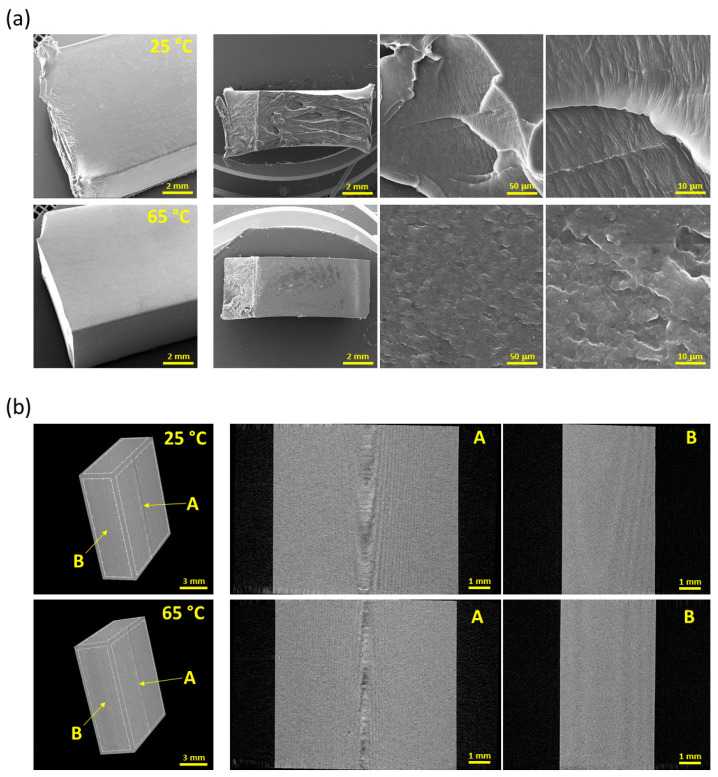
Morphology characterization imaging: SEM images showing the surface morphology of the samples on the left and the fracture morphology following the Charpy impact test at various magnifications on the right−(a) and CT images showing a 3D preview of a scanned sample with the scanning directions marked on the left and images showing sections of the internal structure from two sides (views from direction A and B) perpendicular to each other on the right−(b).
